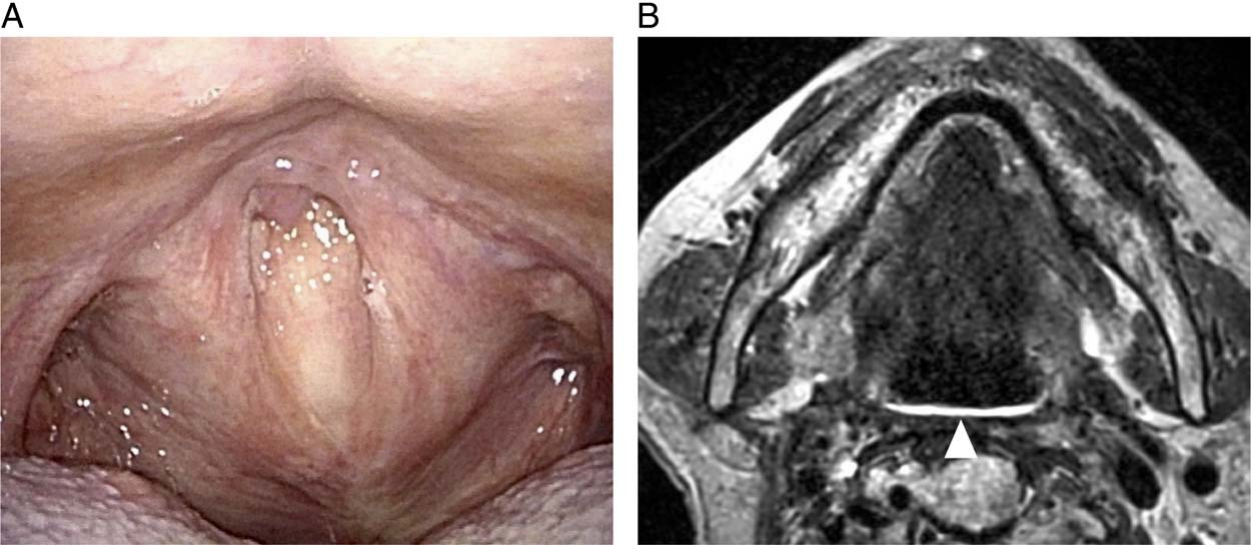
Postoperative images. (A) Endoscopic view. (B) T2-weighted MRI image. Arrowhead represents the place at which the tumor was previously located.
